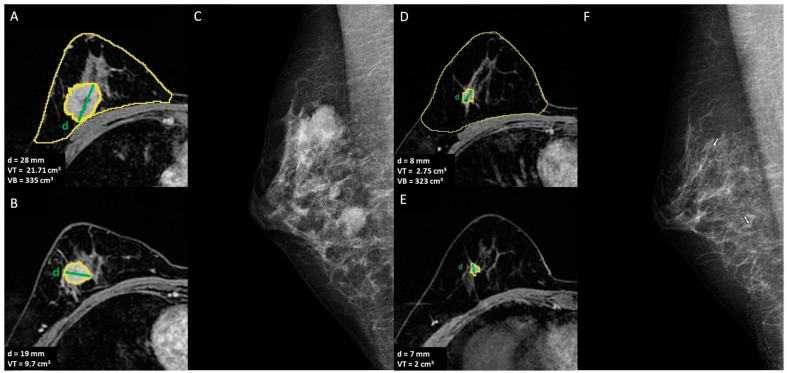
A 42-year-old women with multicentric invasive TN ductal carcinoma with indication of mastectomy after NACT: (A,B) T1-weighted post-contrast MRI to define the extension of the disease and breast volume, VB = breast volume, VT = tumor volume, d = diameter of the lesion; (C) mediolateral oblique mammogram shows the two lesions, (D,E) T1-weighted post-contrast MRI to assess the response to NACT, (F) mediolateral oblique mammogram of the two lesions post-NACT.
